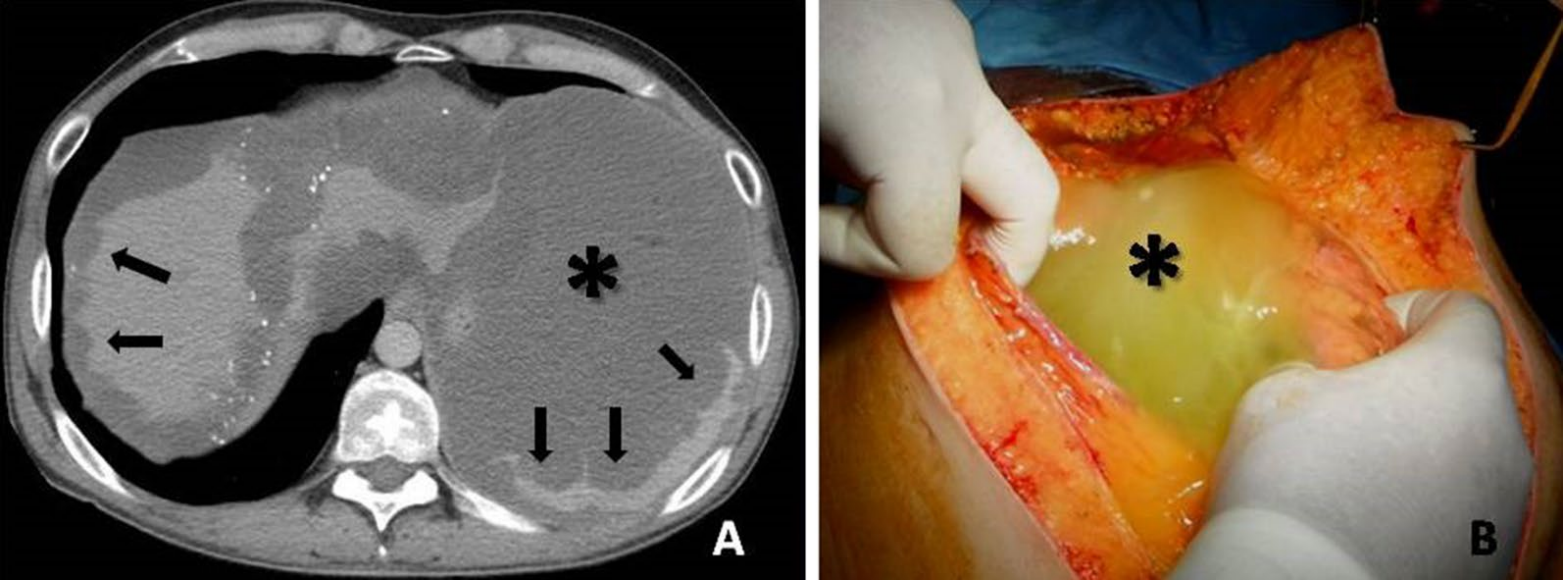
a Contrast-enhanced CT image reveals low-attenuation intraperitoneal gelatinous fluid (asterisk) with mass-like effect and scalloping of the surface of the liver and spleen (arrows). Multiple small calcifications are also present. b Intraoperative photograph shows thick gelatinous fluid filling the peritoneal cavity (asterisk)
